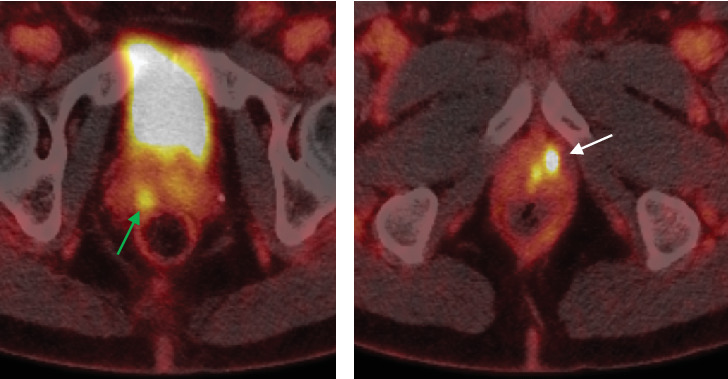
Representative Piflufolastat F-18 PSMA-PET/CT images revealing two PET-avid lesions: (a) right base posterior lesion (green arrow) and (b) left apex anterior lesion (white arrow).
